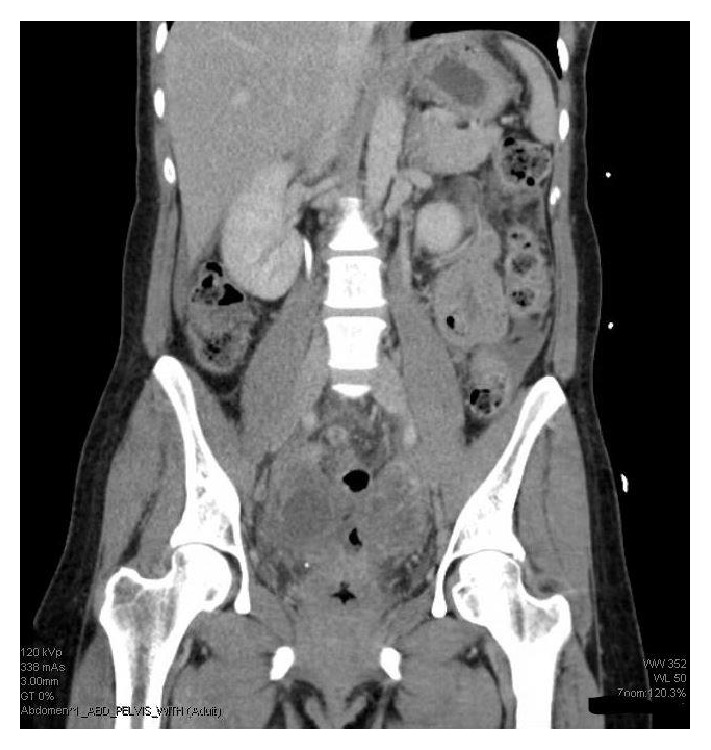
Coronal CT image demonstrating abscess at vaginal cuff.
